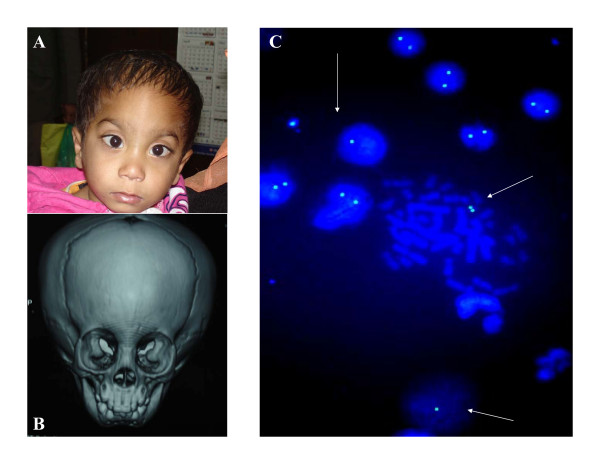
A is showing broad & short nose, small moth, wide philtrum, thin upper lip, hypertelorism, telecanthus, upward slanting almond shaped eyes, low set ears and forehead prominence. B is CT scan of skull showing fusion of metopic suture. C is showing 22q11.2 FISH on lymphocytes (metaphase & interphase cells) without and with deletions (arrow).
